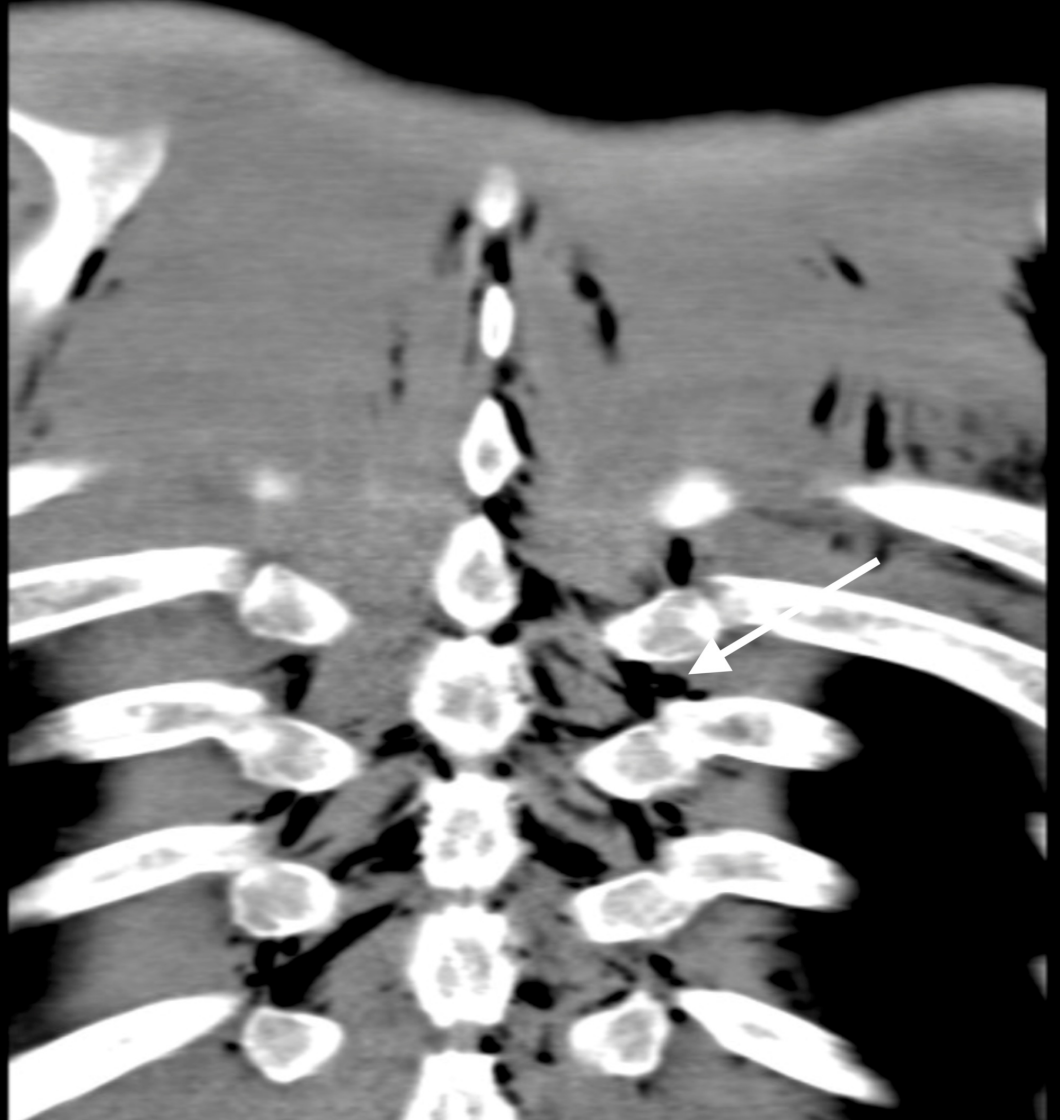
Coronal slice of chest CT highlighting pneumorrhachis and subcutaneous emphysema.
